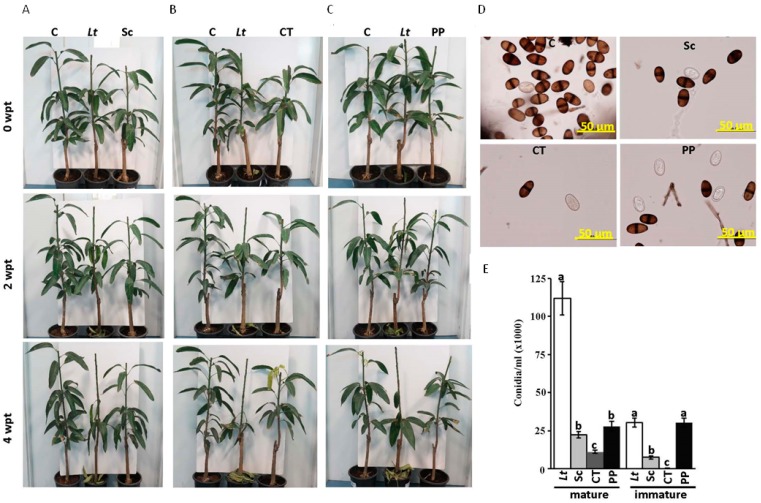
Effect of fungicide treatments on artificially inoculated mango seedlings with *L. theobromae* in the greenhouse. Fungicidal suppression of dieback disease on mango seedlings using (A) Score; (B) Cidely^®^ Top; (C) Penthiopyrad^®^ at 0 (top panel), 2 (middle panel), and 4 (bottom panel) wpt; (D) conidia of the pathogen reisolated from affected tissues of fungicide-treated plants; and (E) the number of conidia/mL at 4 wpt (6 wpi with *L. theobromae*). Seedlings inoculated with *L. theobromae* at two weeks before the fungicide treatment. Values with different letters are significantly different from each other at *p <* 0.05. C, control (non-inoculated seedling); *Lt*, *L. theobromae*-inoculated seedling; Sc, Score^®^; CT, Cidely^®^ Top; PP, Penthiopyrad^®^.
